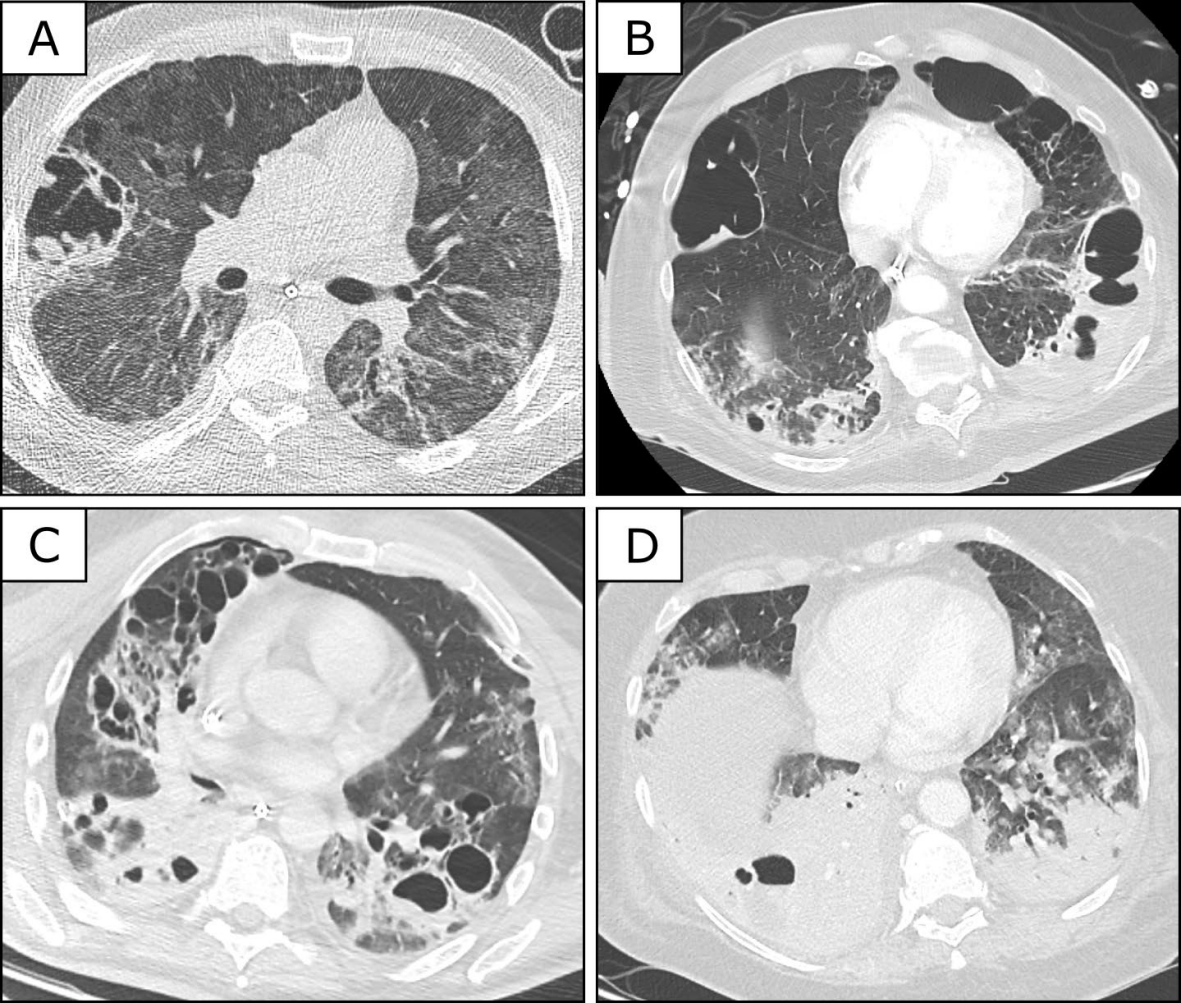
CT scans in COVID-19 patients receiving long term ICU care. CT scans showing typical cavitary lesions in four COVID-19 patients. (A) Single cavity in a 49-year old male patient 29 days after symptom onset; (B) bilateral cavitations in a 77-year old male patient 39 days after onset of symptoms; (C) extensive bilateral cavities in a 57-year old male patient on day 36 after symptom onset; (D) right-sided cavitation in a large lower lobe consolidation in a 69-year old female patient 29 days after onset of symptoms. Only open source software (GIMP 2.10.18 https://www.gimp.org/ and Inkscape, Version 1.0 (4035a4fb49, 2020–05-01) ) were used to generate the figure.
